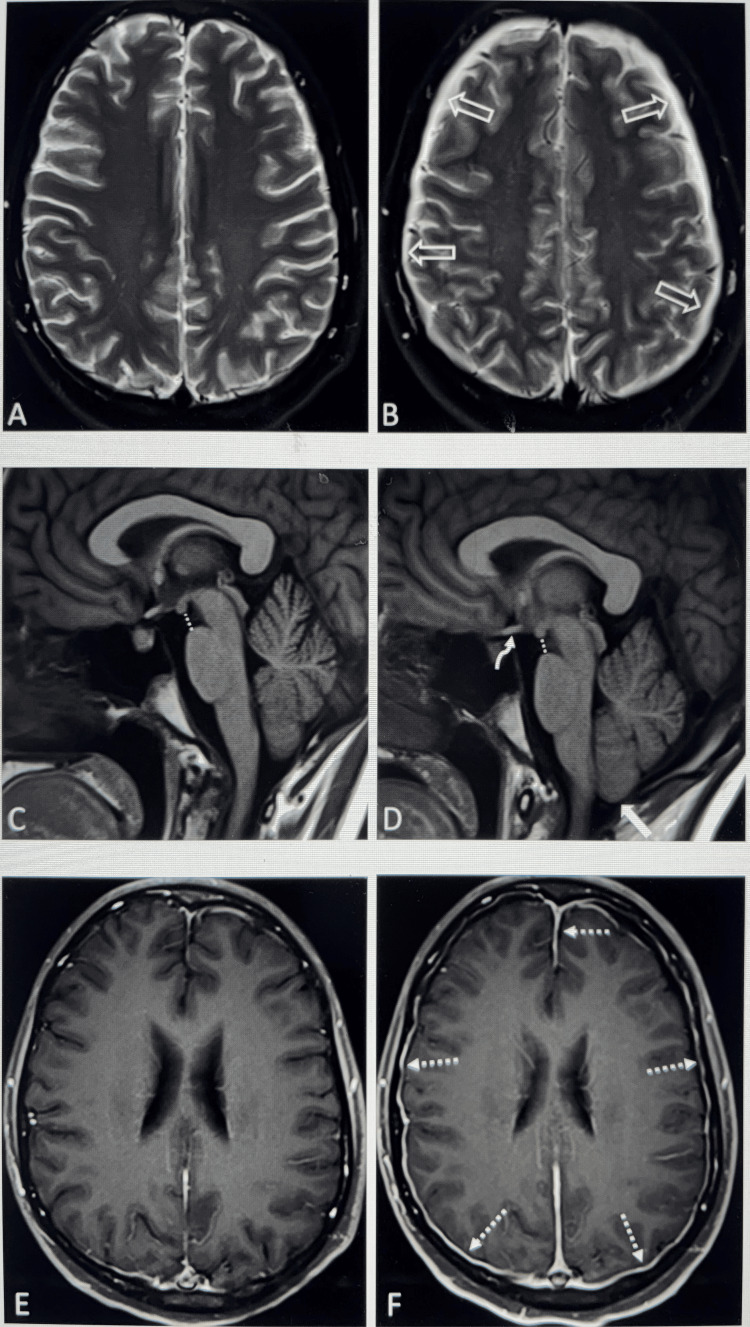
Brain MRI (Patient 1) Patient 1 with successive brain MRIs after repeated lumbar punctures and worsening post-dural puncture headaches, showing new intracranial findings suggesting intracranial hypotension and CSF leak. Patient 1 had four LPs before EBP. After the second lumbar puncture, an MRI was obtained for a mild to moderate headache (A, C, E), showing a normal T2-weighted image (A). After two more lumbar punctures, an MRI was repeated 10 days after the first MRI due to worsening headaches (B, D, F), with new bilateral subdural fluid collections (B, open white arrows). Baseline sagittal T1-weighted images show normal position of the cerebellar tonsils and normal mamillopontine distance (C, dotted line). Follow-up sagittal T1 (D) shows subtle narrowing of the mamillopontine distance (dotted line), inferiorly positioned cerebellar tonsils (solid arrow), and drooping optic chiasm (curved arrow). Baseline axial post-contrast T1 turbo spin echo images (E) show no abnormal enhancement, with abnormal pachymeningeal enhancement bilaterally on the follow-up 10 days after (F, dotted arrows). CSF: cerebrospinal fluid; LP: lumbar puncture; EBP: epidural blood patch
